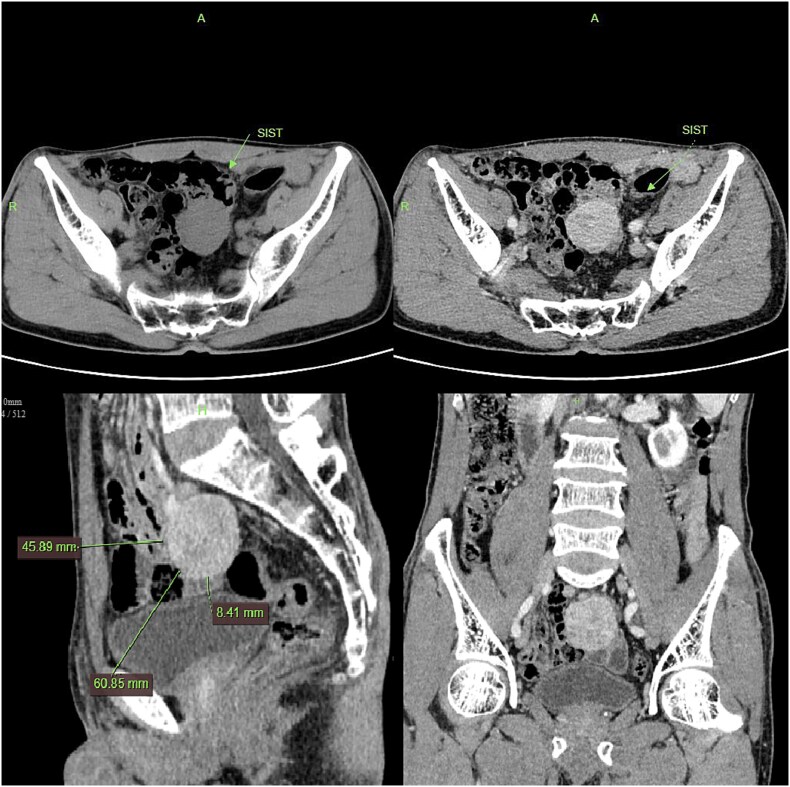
CT imaging shows a pelvic lesion with soft tissue density on non-enhanced scans, marked enhancement of the primary tumor mass after contrast, and coronal/sagittal reconstructions delineating its anatomical location.
